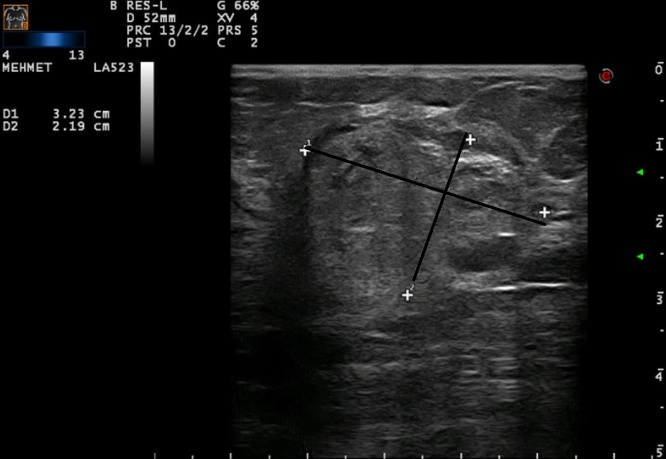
On sonographic imaging the lesion had smooth contours and was hypoechogenic with large hyperechoic components in between.
